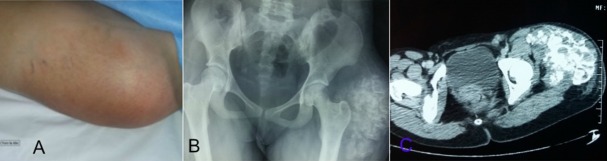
A) tuméfaction de la face antero-externe de la hanche gauche arrivant jusque au 1/3 supero-externe de la cuisse; B) radiographie du bassin en incidence de face montrant des opacités calcifiées, séparées par des septas radio-transparents péri-articulaire au niveau de la hanche gauche; C) tomodensitométrie montrant une masse en regard de l’articulation coxo-fémorale gauche, de densité calcique, bourgeonnante rappelant un aspect en chou-fleur
